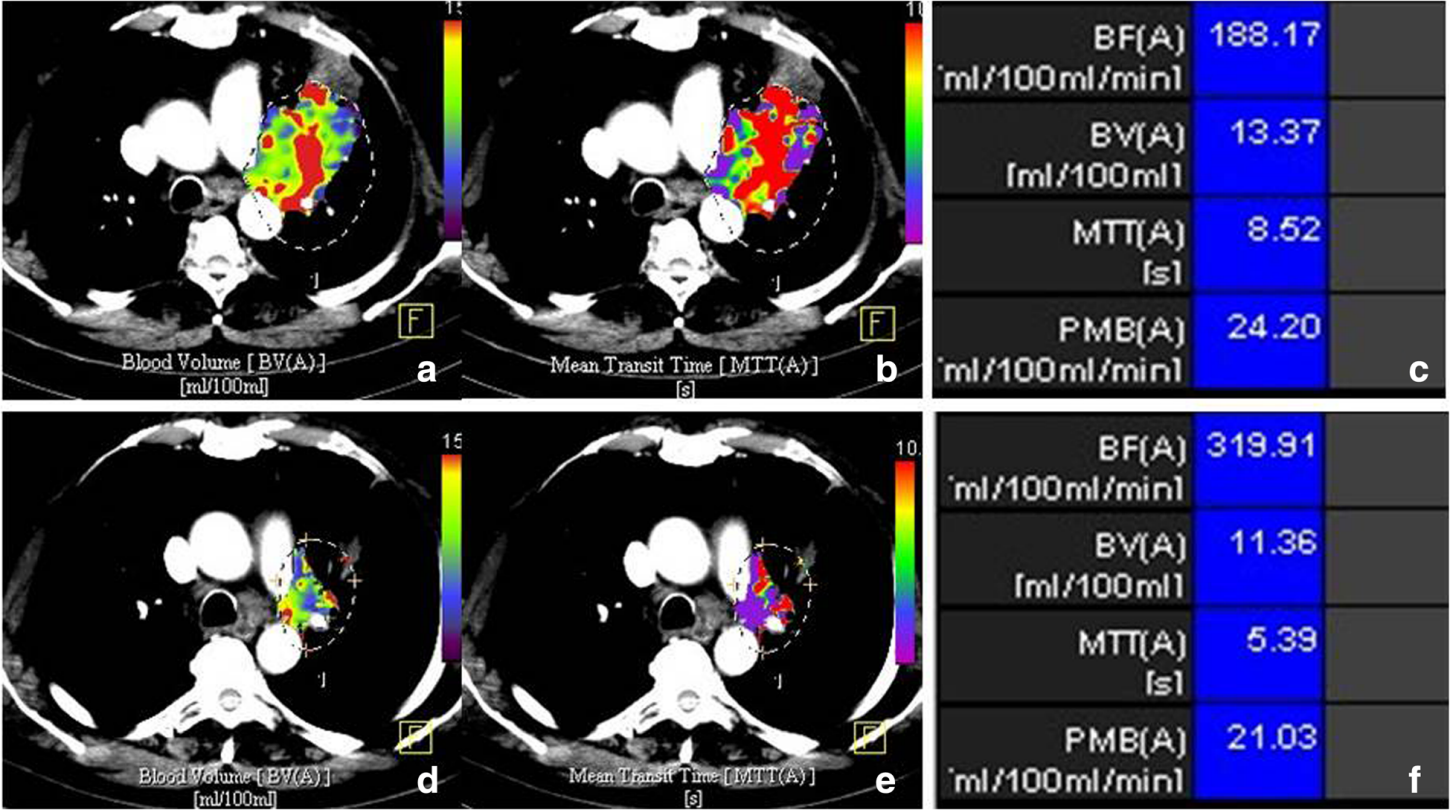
Perfusion computed tomography of left upper lobe epidermoid carcinoma. Colour coded maps and quantitative values of blood volume and mean transit time before (a, b, c) and after (d, e, f) platinum derivatives treatment show a decrease in these two perfusion parameters after treatment. *BF* Blood flow, *BV* Blood volume, *MTT* Mean transit time, *PMB* Permeability
